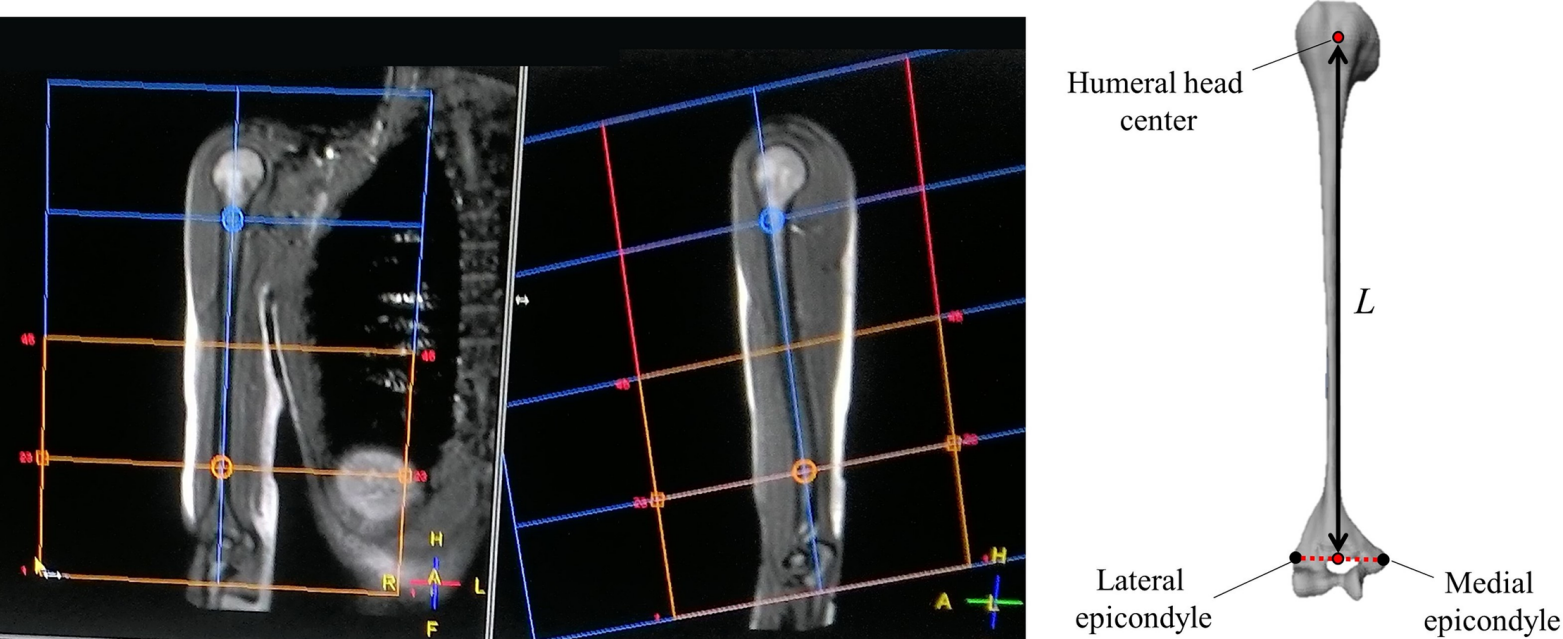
Magnetic resonance imaging (MRI)-based humerus length measurement. Two MRI slices on the frontal plane are shown on the left-hand side, and the 3D reconstruction of the humeral bone is shown on the right-hand side. The reference humerus length value *L* is obtained as the distance between the humeral head centre and the midpoint between the two distal epicondyles of the reconstructed humeral bone.
